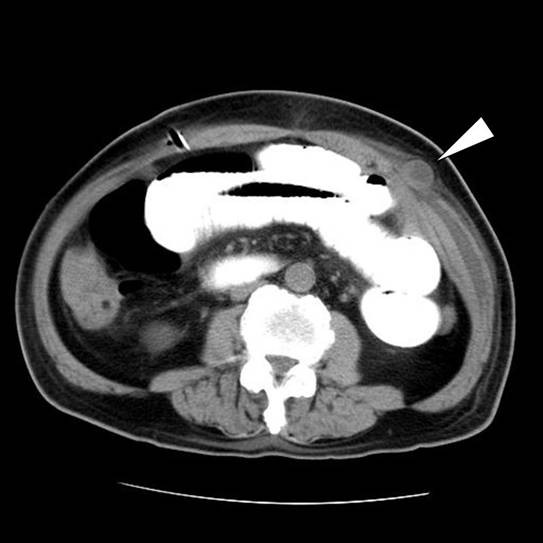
Computed tomography (CT) shows the enlarged duodenum and a mass lesion protruding into the muscular layer of the abdominal wall (arrowhead).
